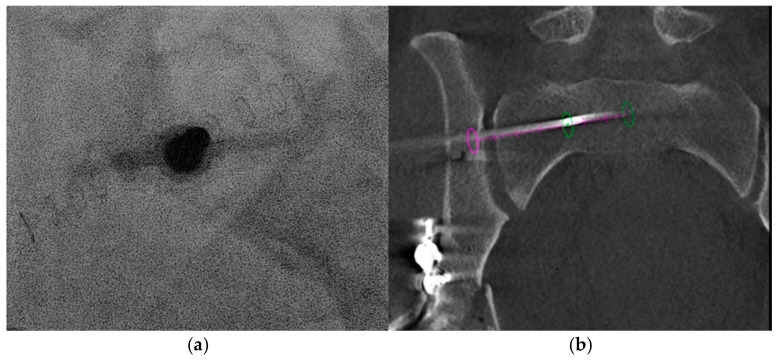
Fluoroscopy and CBCT guidance during the procedure. (a). Fluoroscopic guidance showing trocar positioning at a bull’s eye view at the level of the first sacral segment (S1). (b). Coronal view with CBCT guidance. The pin is visible in place, with its distal end reaching the promontory. The purple circle indicates the iliac entry point, while the green circle marks the target at the sacral promontory. The display represents the progression view.
